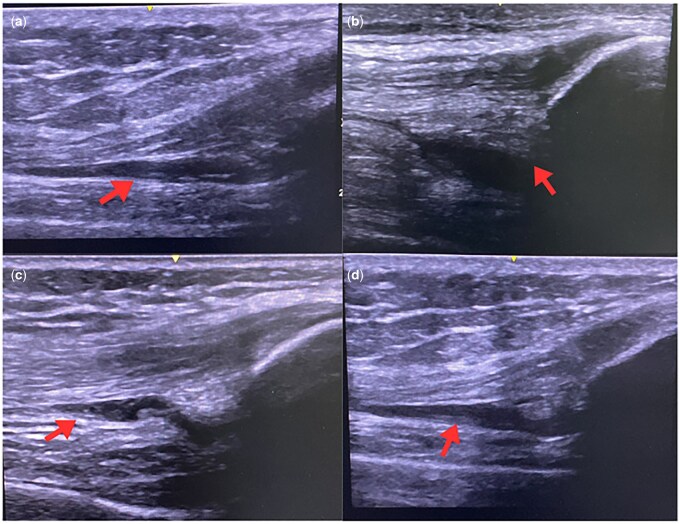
Ultrasonographic images of knee synovial effusion in patients. Images were obtained with the knee in a neutral position and the probe positioned longitudinally over the suprapatellar region. Arrows in a and c indicate synovial effusion with synovial hypertrophy, b highlights the displaced prepatellar fat pad, and d demonstrates synovial hypertrophy
